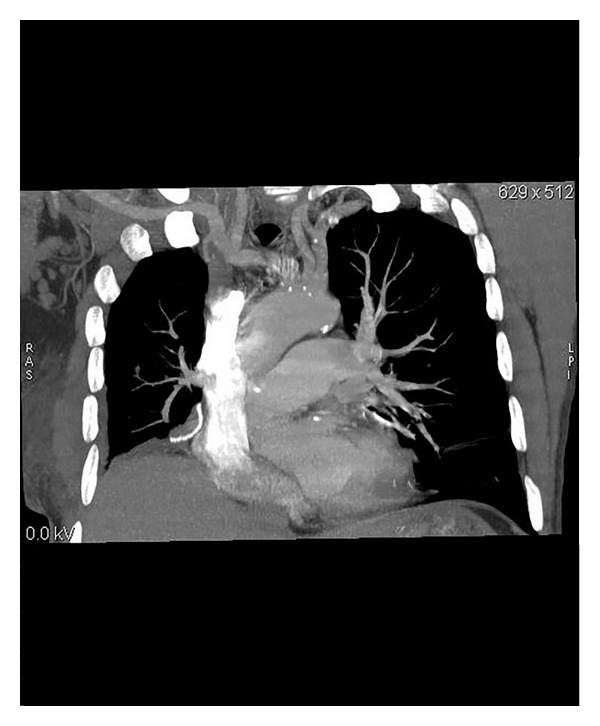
Another coronal oblique CT image denoted a PAPVC from the right upper lobe to the superior vena cava (arrow).
